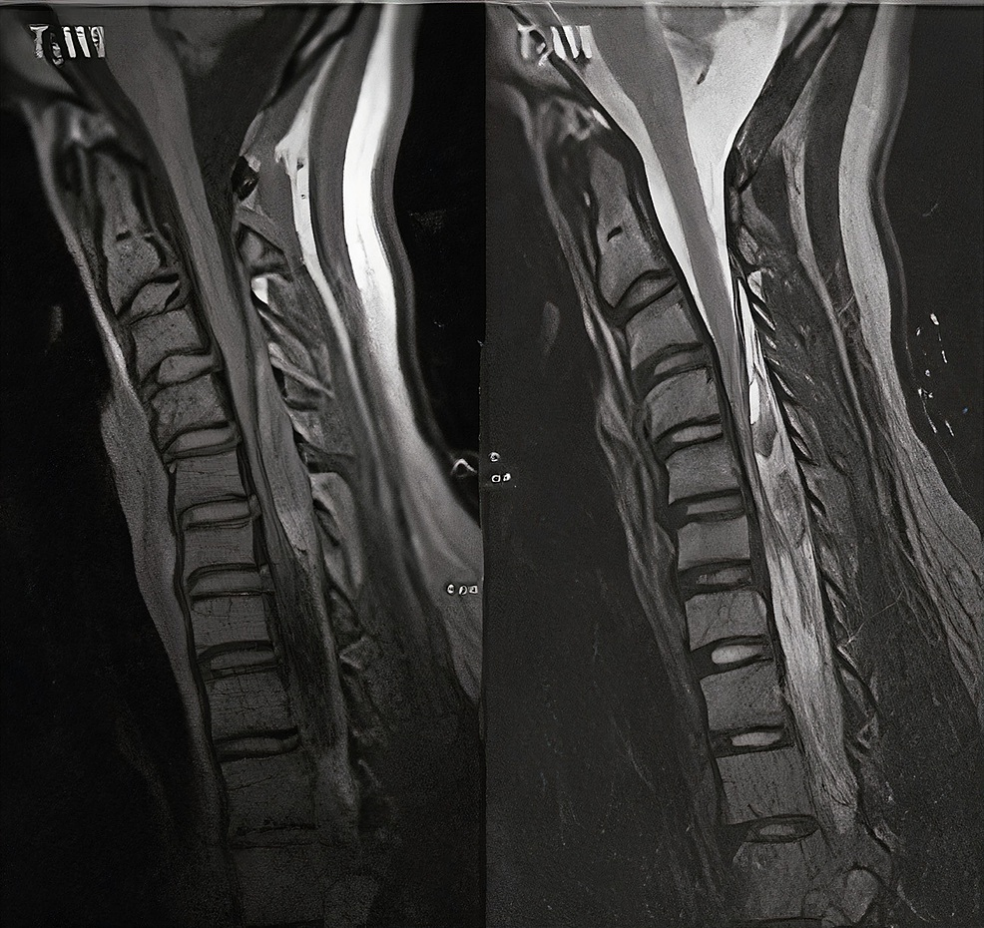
Sagittal MR images in T1WI & T2WI A heterogenous fusiform long left posterolateral epidural lesion that appears generally isointense on T1W, and heterogeneously high on T2W images with focal areas of altered signal within. No remarkable contrast enhancement may could be seen. Findings are in keeping with epidural hematoma.
